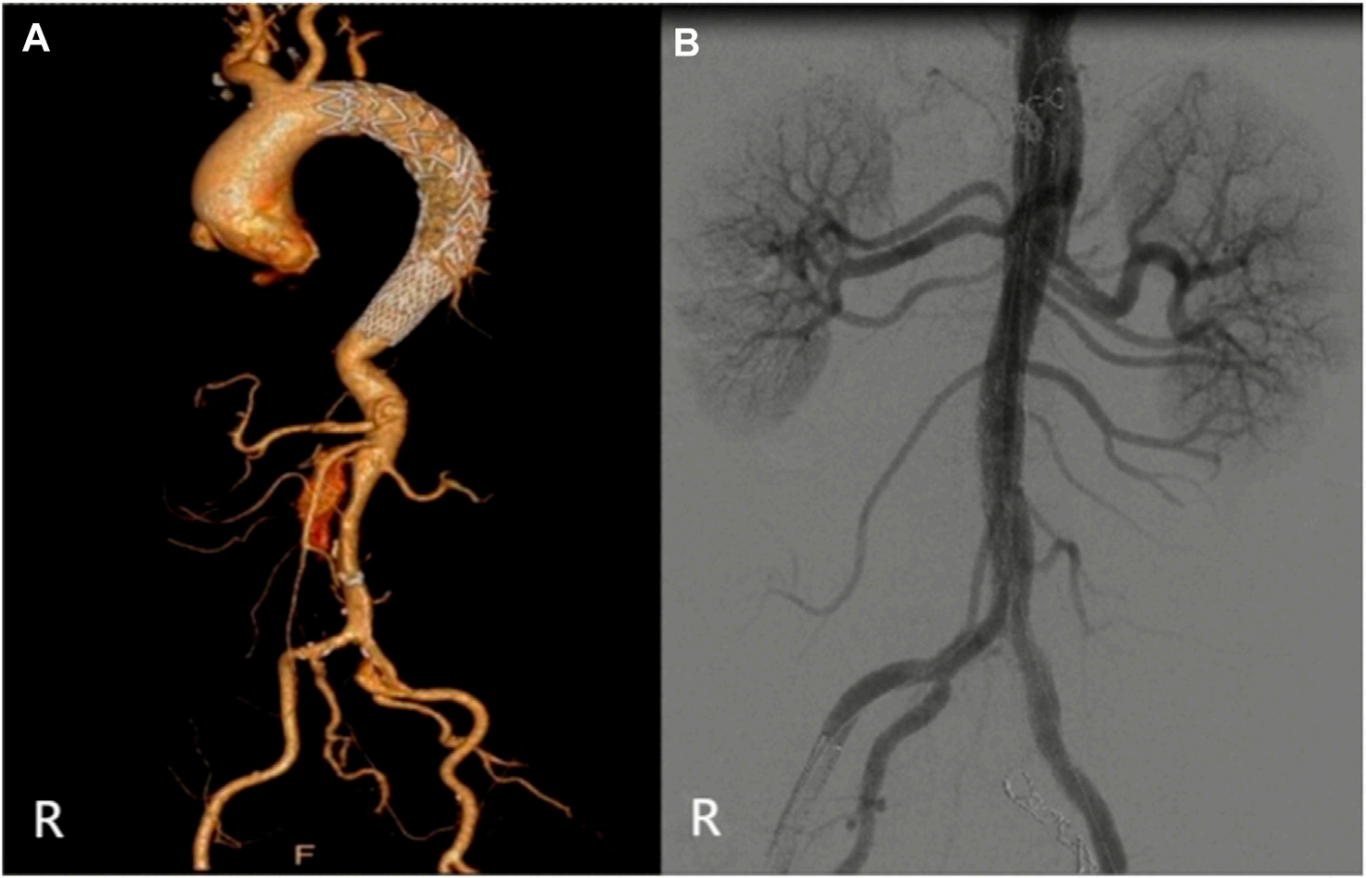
(Case 3) (A) Preoperative CTA. (B) Intraoperative imaging.
